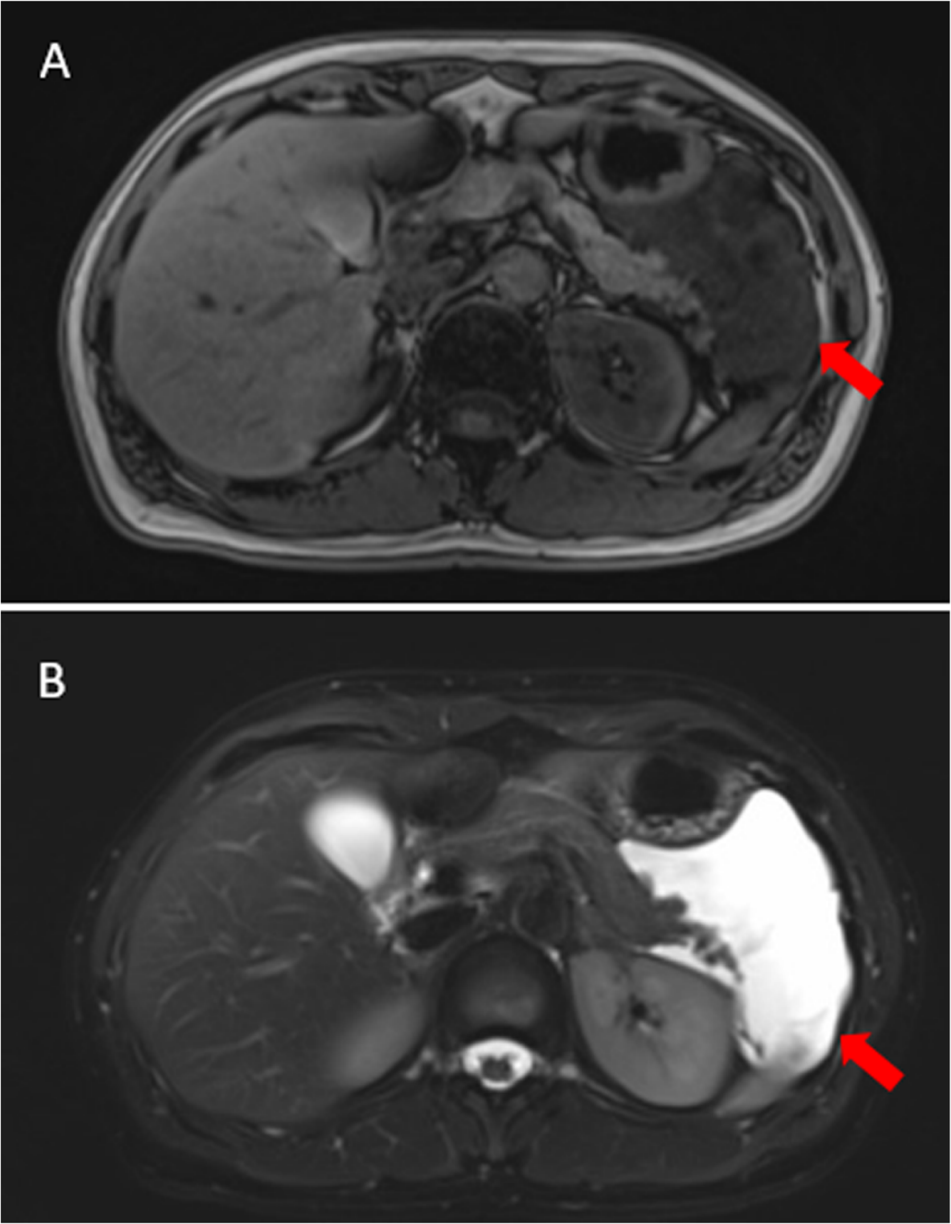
MRI of Chinese adult patients with ACL. A. Low signal in T1WI sequence of MRI of case no. 3 (red arrow). B. High signal in T2WI sequence of MRI of case no. 3 (red arrow). ACL, abdominal cystic lymphangioma; MRI, magnetic resonance imaging; T1WI, T1-weighted image; T2WI, T2-weighted image
